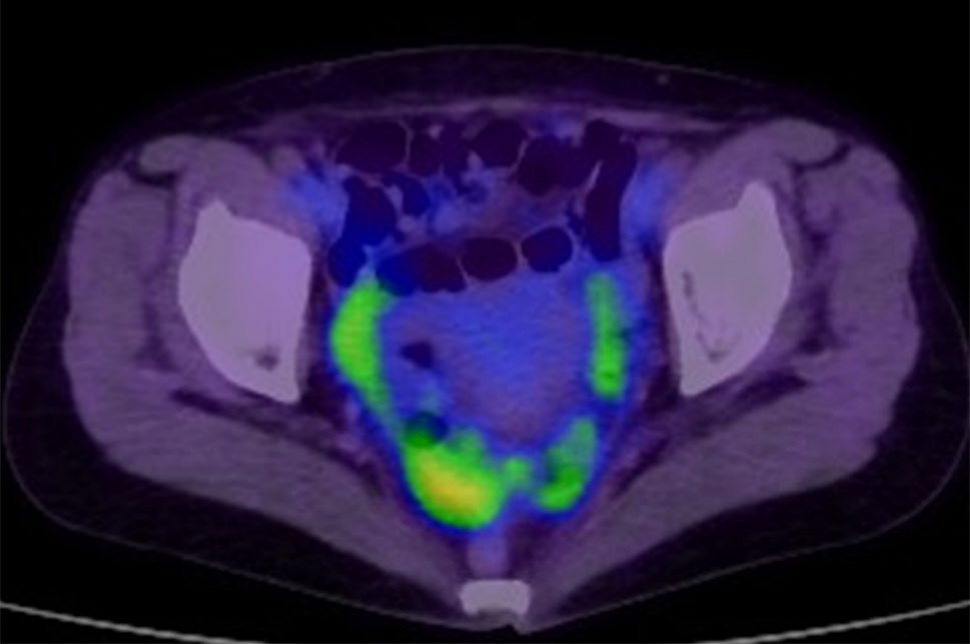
FDG-PET/CT shows slight FDG accumulation in the pelvic peritoneum, suggesting peritoneal dissemination of breast cancer
